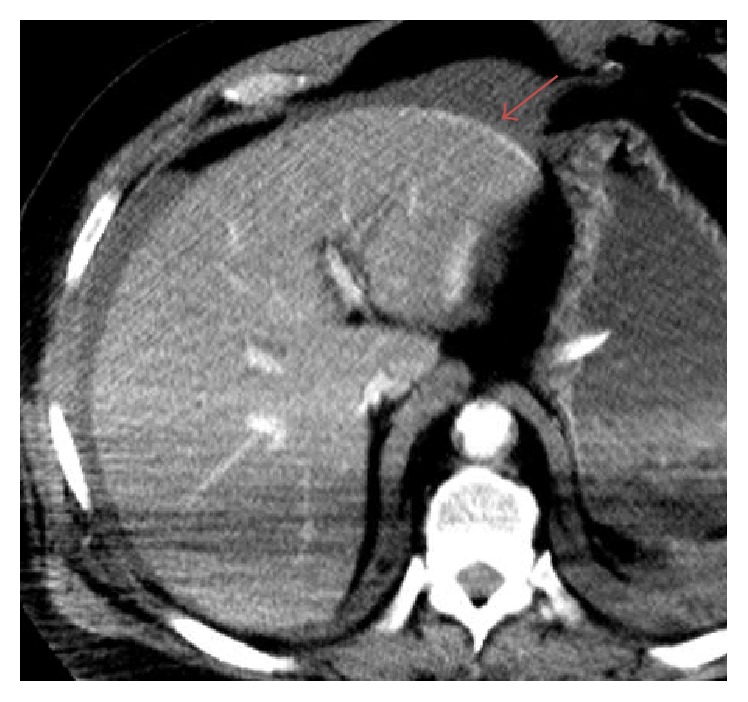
64-year-old male diagnosed with traumatic right ventricular rupture. Routine axial contrast enhanced CT demonstrates extravasated contrast communicating with the intraperitoneal space and lining the liver (arrow).
